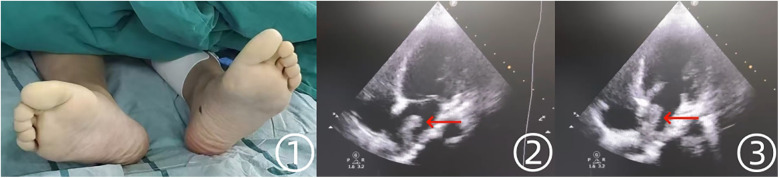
Preoperative findings of the patient's lower extremities and echocardiographic manifestations. ① The patient's bilateral lower extremities appeared pale and cold preoperatively. ②, ③ Echocardiography revealed a hyperechoic mass in the left atrium.
